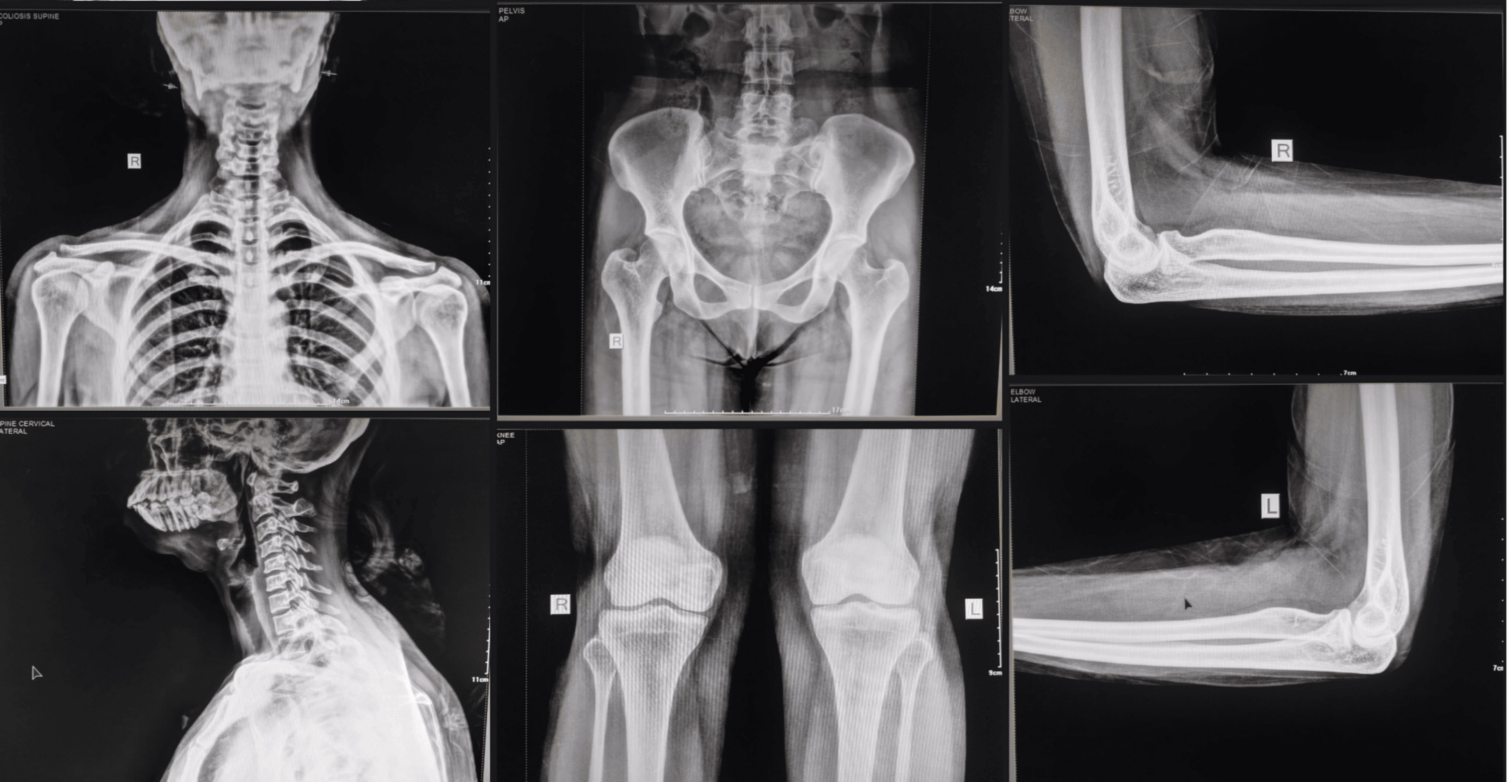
Plain chest X-ray AP view and lateral view. Plain X-ray of the right and left pelvis with the proximal part of the right and left femur AP view; plain X-ray of right and left knee showing distal part of femur and proximal part of the tibia and fibula AP view. Plain X-ray of the right and left elbow showing the distal part of the humerus and the proximal part of the radius and ulna, lateral view AP view: anteroposterior view; R: right; L: left
